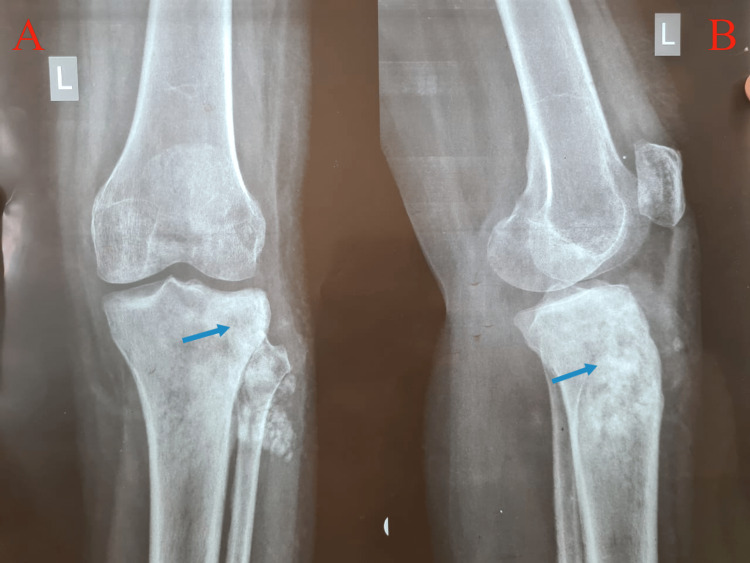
X-ray of the knee at the time of presentation. (A) Anteroposterior and (B) lateral view showing osteolytic lesion with periosteal reaction and tumour surrounding in the proximal 1/3 of the shaft of the tibia (blue arrows). Figure credits: Harshika Kaur Saluja (author).
